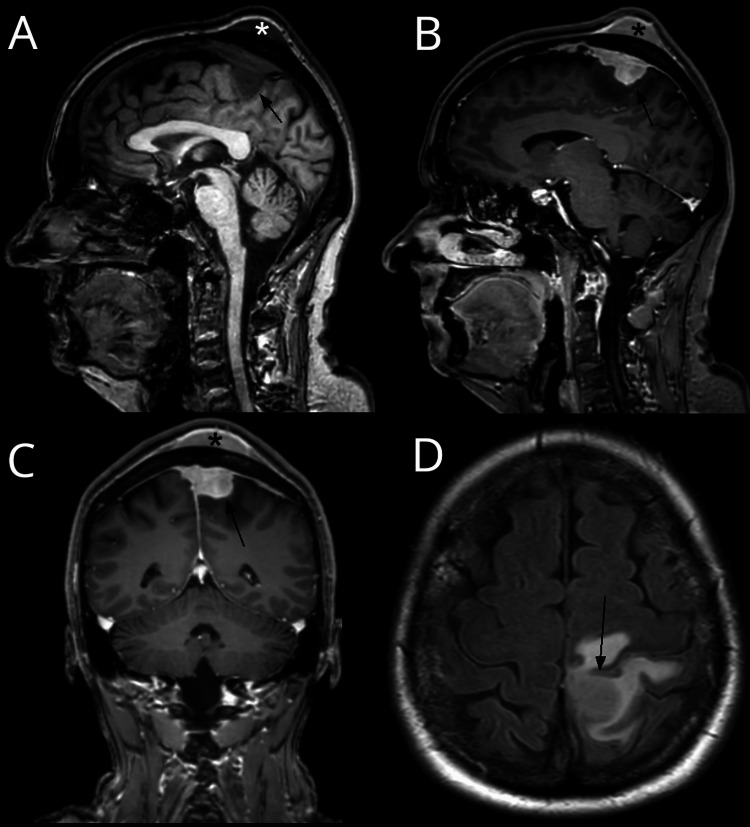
Cranial MRI without and with contrast medium. Sagittal T1-weighted MRI without (A) and with contrast medium (B), as well as coronal T1-weighted MRI with contrast medium showing extra- and intracranial tumor mass (asterisk and arrow respectively), which are hypointense and homogenously contrast enhancing (C). Axial FLAIR-MRI showing the tumor with surrounding edema (D).
